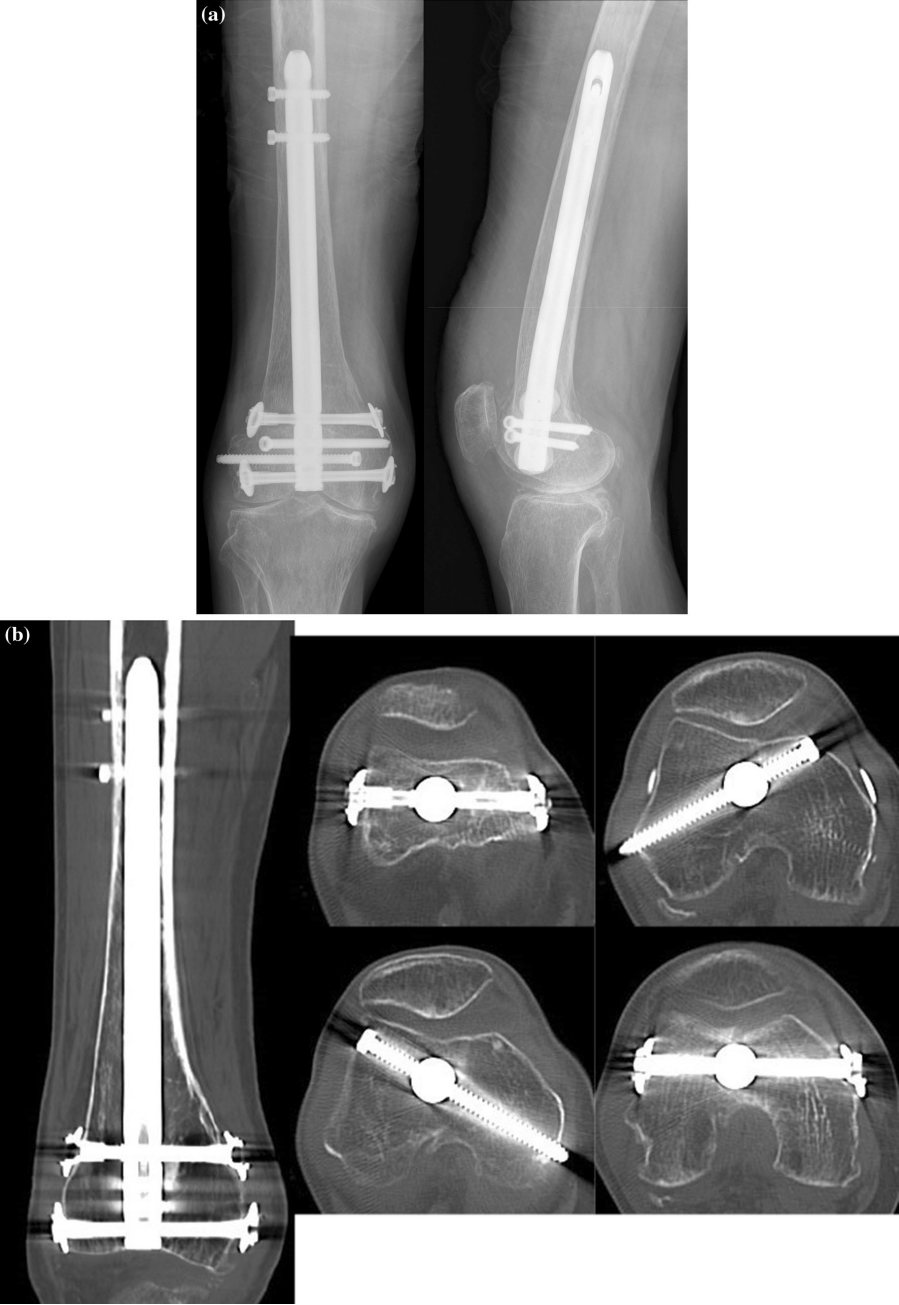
a Radiographs demonstrating bony union and no loss of reduction. b Computed tomography demonstrating bony union and suitable congruity
